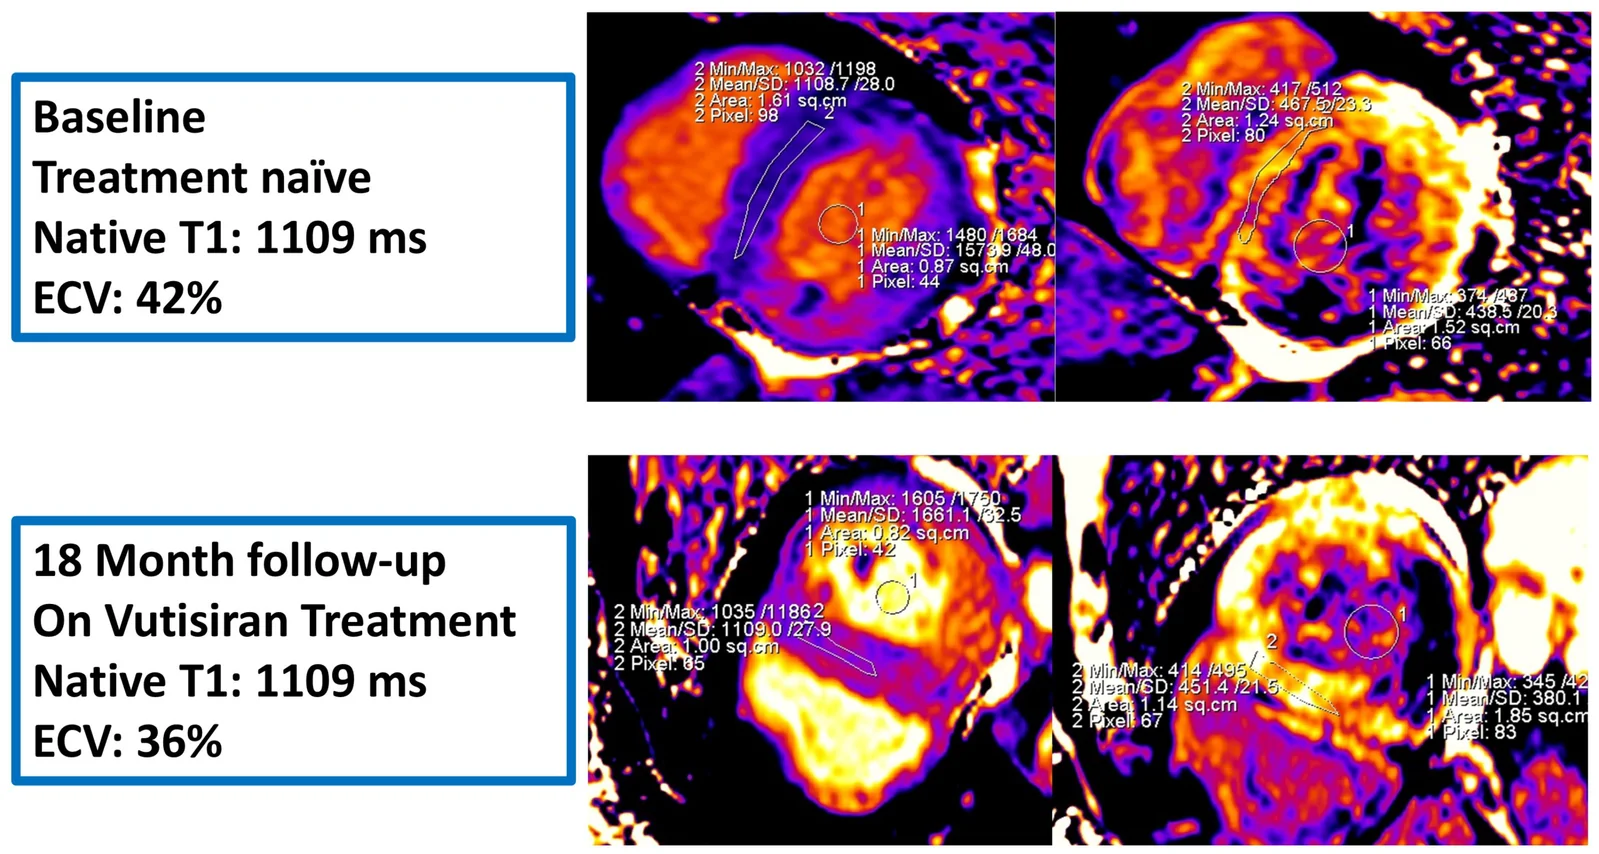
Cardiac magnetic resonance imaging of the same patient reported in Figure 2. Short-axis, mid-slice, at baseline without treatment (Naïve) (top panels, from left to right: native T1 and post-contrast T1 mapping) and 18-month follow-up after initiation of vutrisiran therapy (bottom panels, from left to right: native T1 and post-contrast T1 mapping) in a patient with TTR cardiac amyloidosis. At follow-up, a regression of ECV is observed.
